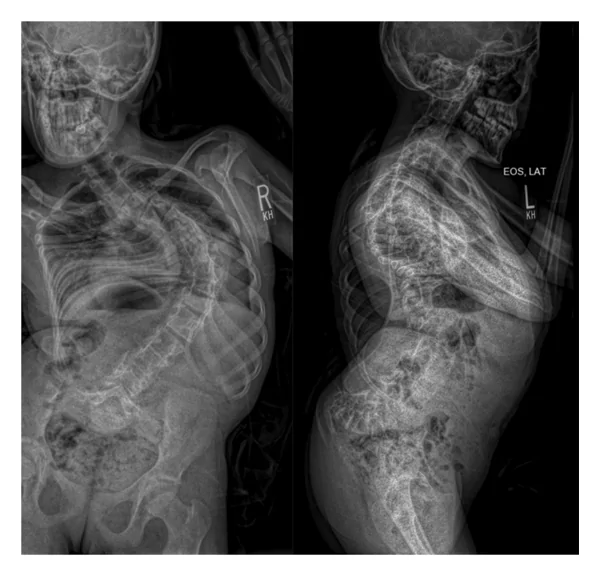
Preoperative radiographs, coronal and sagittal views showing significant thoracic spinal deformity.
